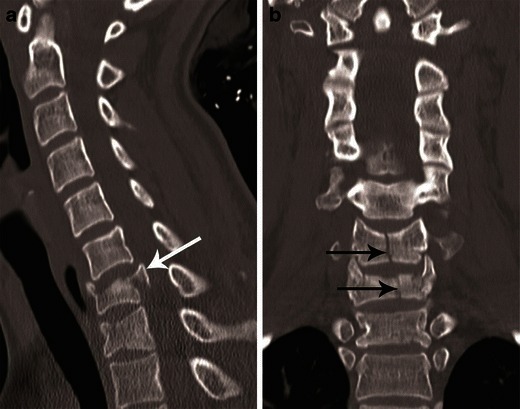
Burst fracture. a Mid-sagittal CT image showing a comminuted fracture of C7 with nearly symmetrical loss of vertebral body height with extension of the fracture to the posterior cortex and retropulsion of fragment from the posterospuerior cortex of C7 (*white arrow*)—suggestive of a burst fracture. Vertebral body comminution is better seen on axial images as shown in Fig. 9b. b Coronal CT image showing a vertical split fracture of C6 and C7 (*black arrows*). These fractures are oriented in the sagittal plane, hence best seen on coronal images and highly suggestive of axial loading injury
